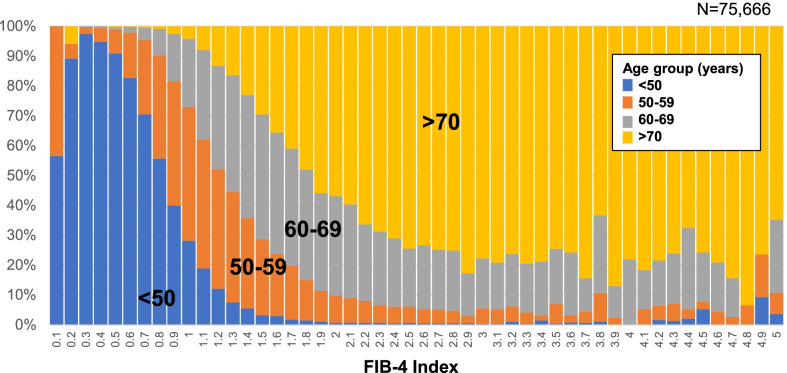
Proportion of age group in each FIB-4 index value in residents who underwent abdominal ultrasonography at health checkups in Japan (N = 75,666). This figure shows the proportion of age group in each FIB-4 index value in residents who underwent ultrasonography at health checkups in Japan. Blue color represents the age group under 50, orange represents the age group 50–59, gray represents the age group 60–69, and yellow represents the age group over 70. Most of the high FIB-4 values are in the 70 + age group
